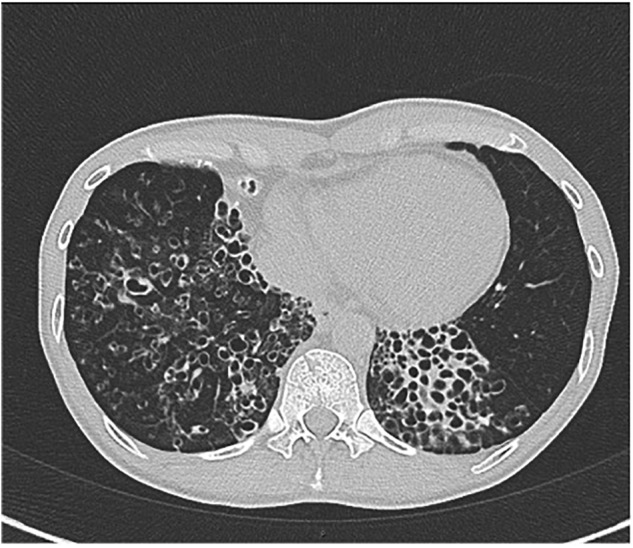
CT chest image showing cystic bronchiectasis in the right and left lower lobes.
